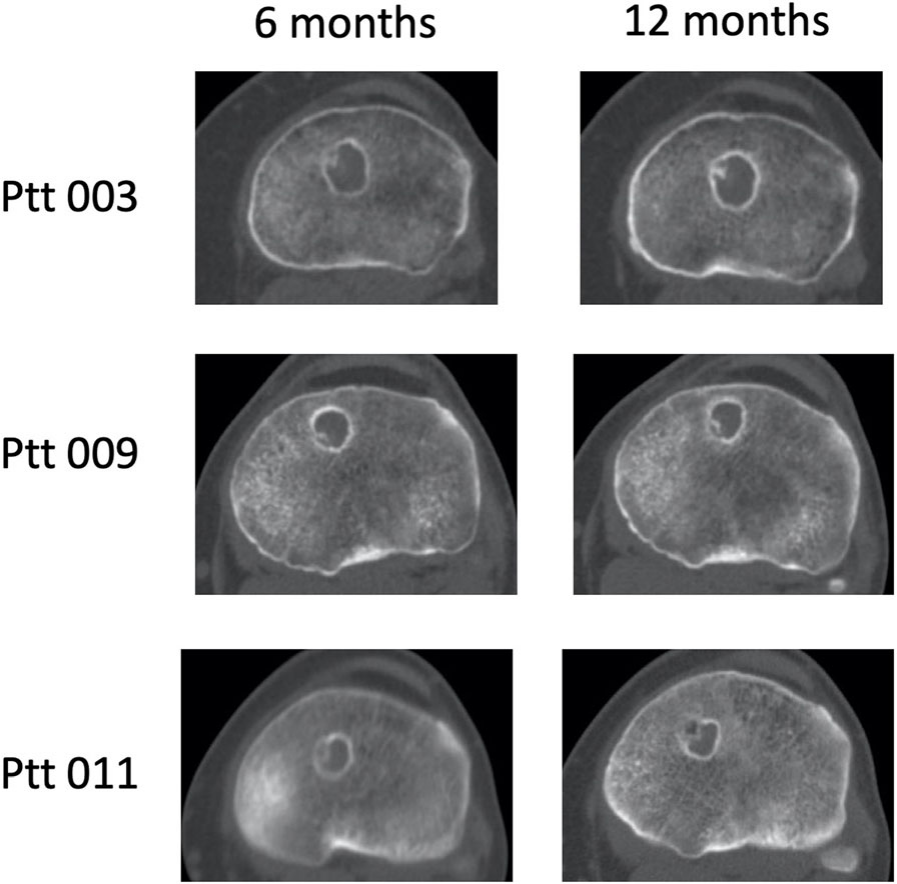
CT scanning images of characteristic bone ingrowth pattern. Three examples of average ingrowth at 6 and 12 months follow-up are shown
